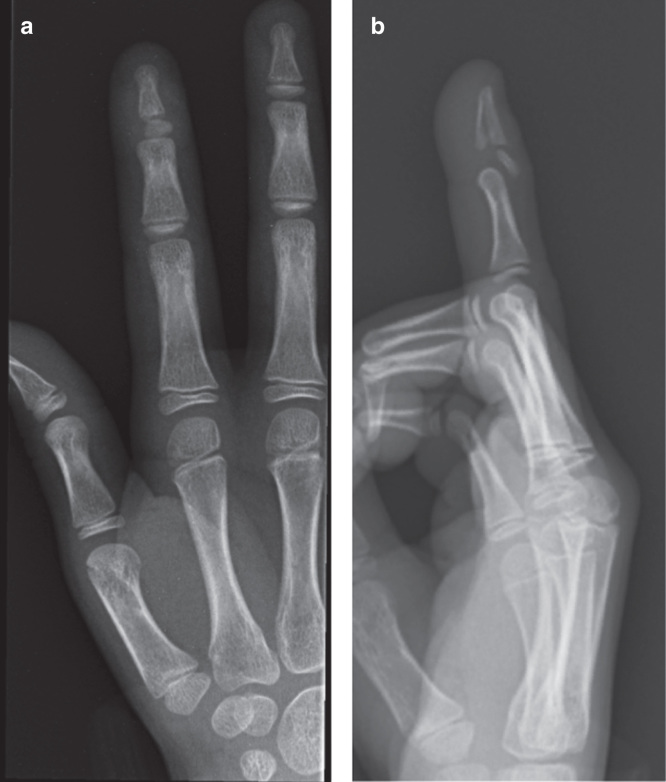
Anteroposterior and lateral radiographs demonstrating a Salter–Harris Type 1 fracture to the right index finger distal phalanx.
